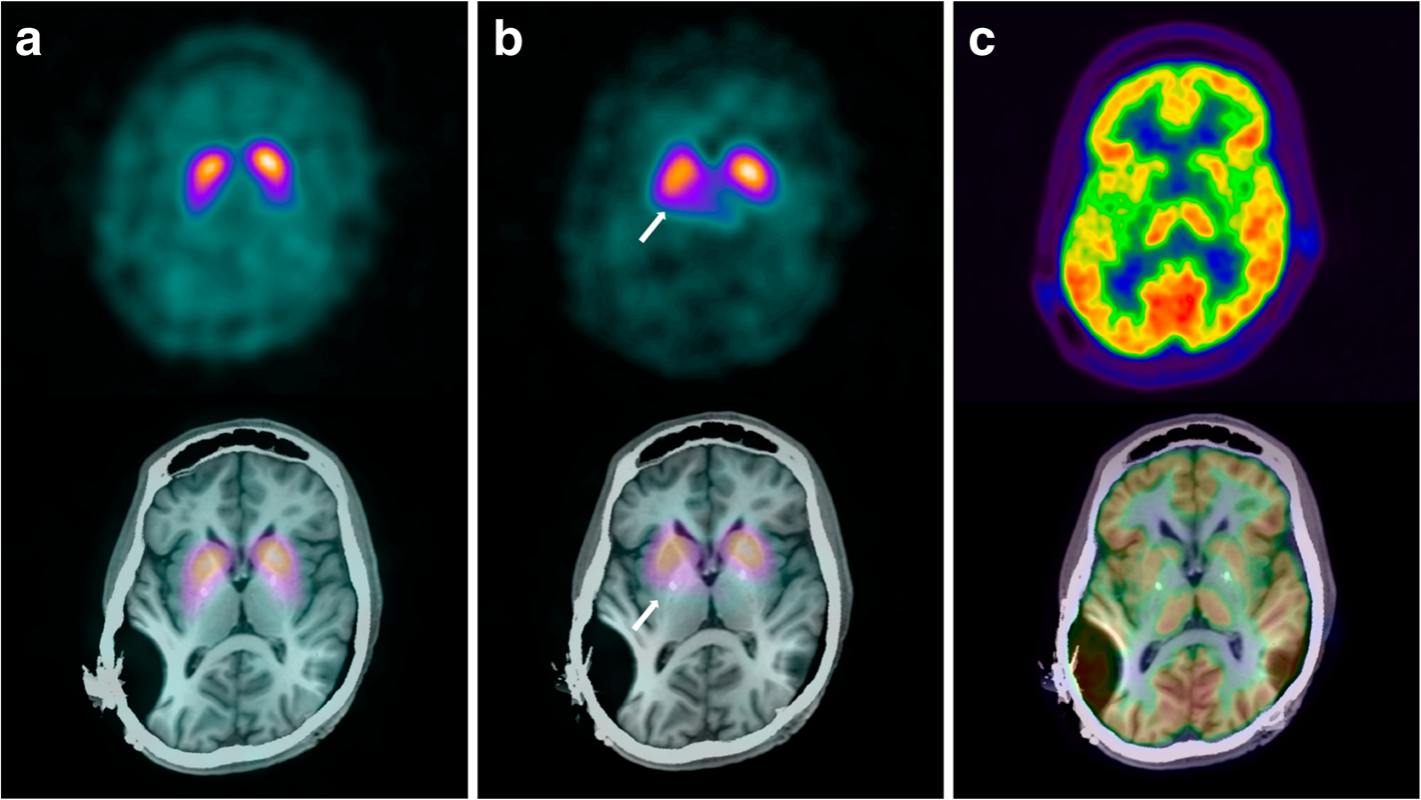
Brain radiotracer imaging of pre- and postsynaptic striatal dopamine transmission and glucose uptake. Upper images show emissions only, and lower images are fused with MRI (T1 series, 2013). a
